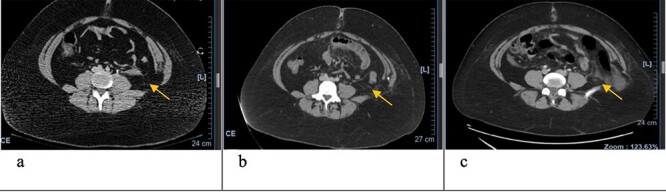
Axial CT scan with arrows pointing to internal and external oblique defects in June (a), August (b) and September (c); panel (a) shows rupture between internal and external oblique muscles at the attachment point of the quadratus lumborum; panel (b) shows fat stranding, suggesting inflammation of the epiploic appendage, and may be an early sign of delayed traumatic wall hernia; panel (c) shows herniation through the muscular defect.
